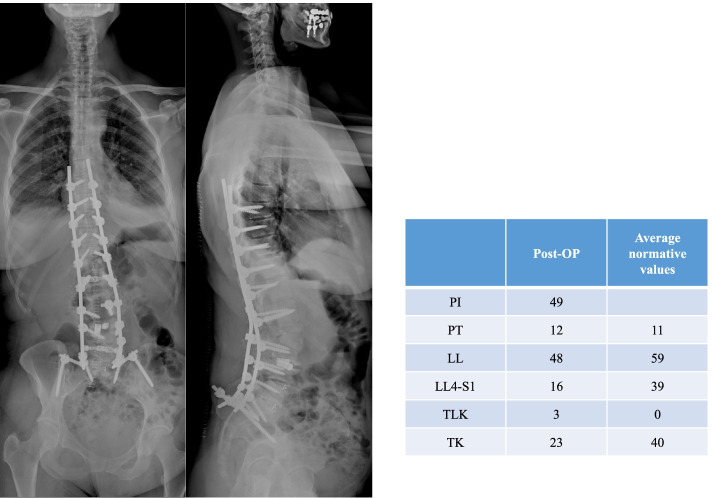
Postoperative x-ray following revision surgery in December 2012. A T8-pelvis instrumentation with a pedicle subtraction osteotomy in L2 and a TLIF L4/5 and L5/S1 was performed. The revision allowed correction of the DJK and restoration of the sagittal profile to values approaching the normative ones (*PI* Pelvic incidence, *PT* Pelvic tilt, *LL* Lumbar lordosis L1-S1, *LLL* Low lumbar lordosis L4-S1, *TLK* Thoracolumbar kyphosis T10-L2, *TK* Thoracic kyphosis T1-T12; all measurements are in degrees)
